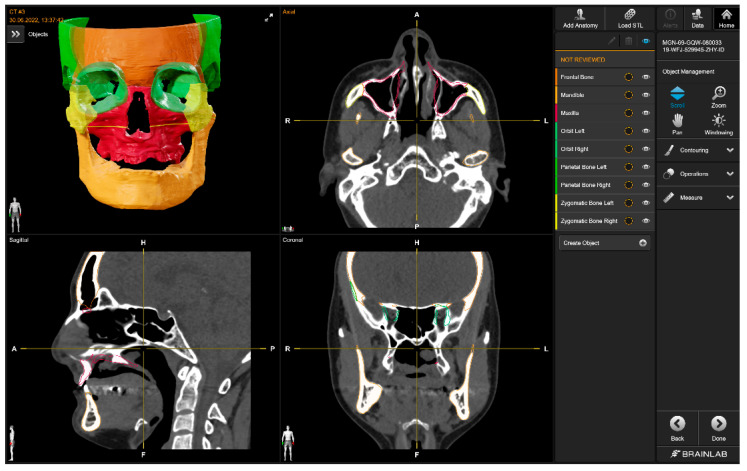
Brainlab’s user interface (CT w/A 1 displayed).
